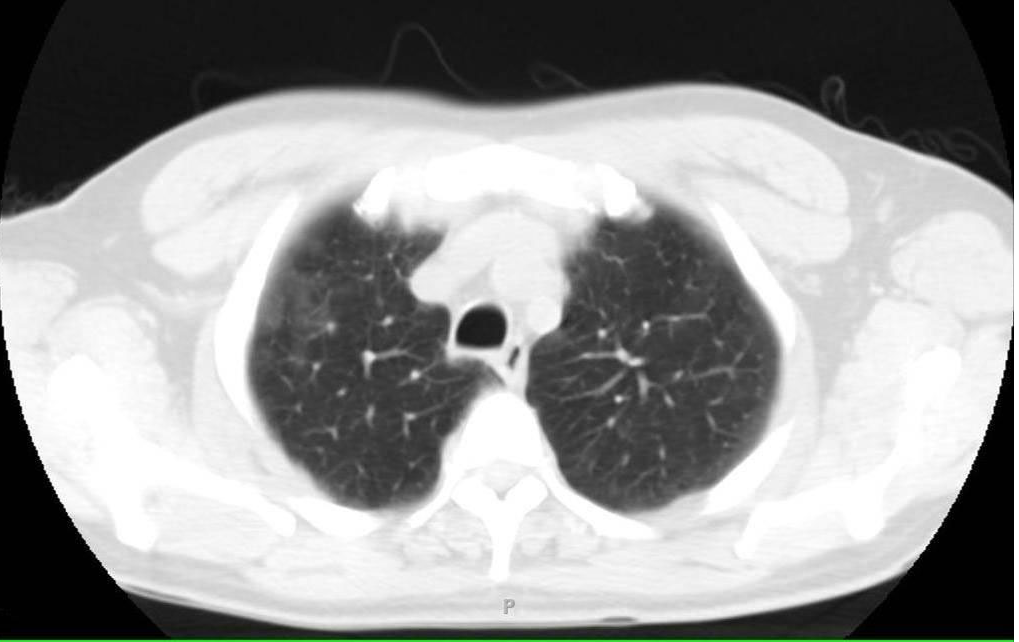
CT scan showing subtle right middle lobe and right upper lobe ground-glass opacity.
